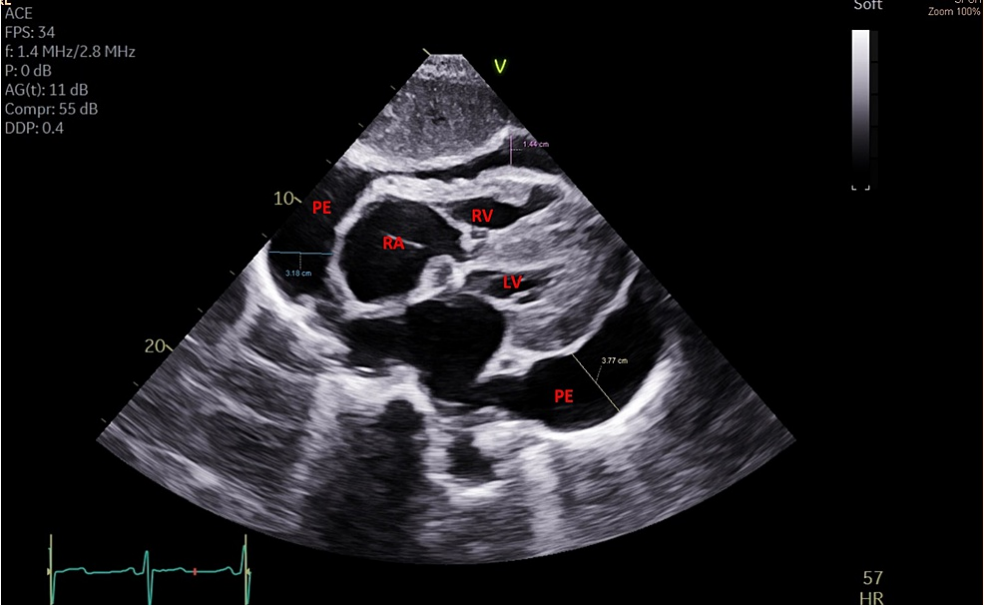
Transthoracic echocardiogram (TTE) subcostal view A large circumferential pericardial effusion with early diastolic right ventricle free-wall inversion. LV (Left ventricle), PE (Pericardial effusion), RA (Right atrium), RV (Right ventricle).
